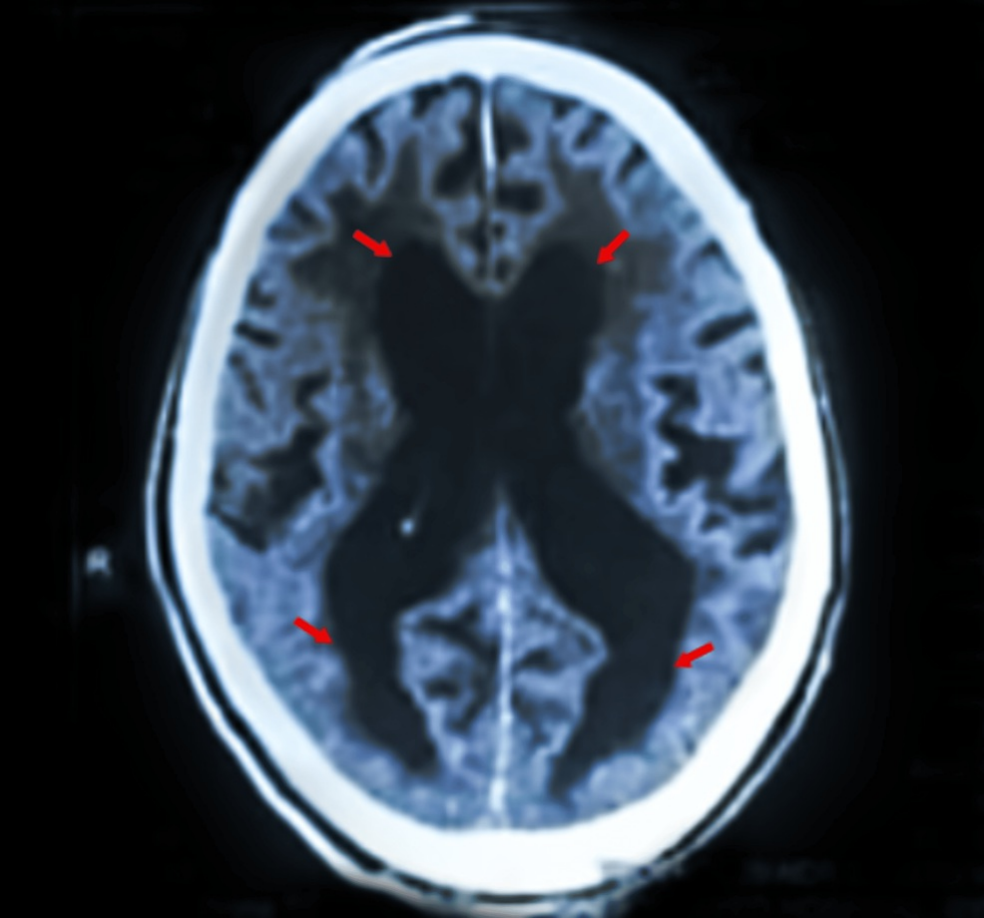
CT scan of the brain showing dilation of the lateral ventricles (arrows).
